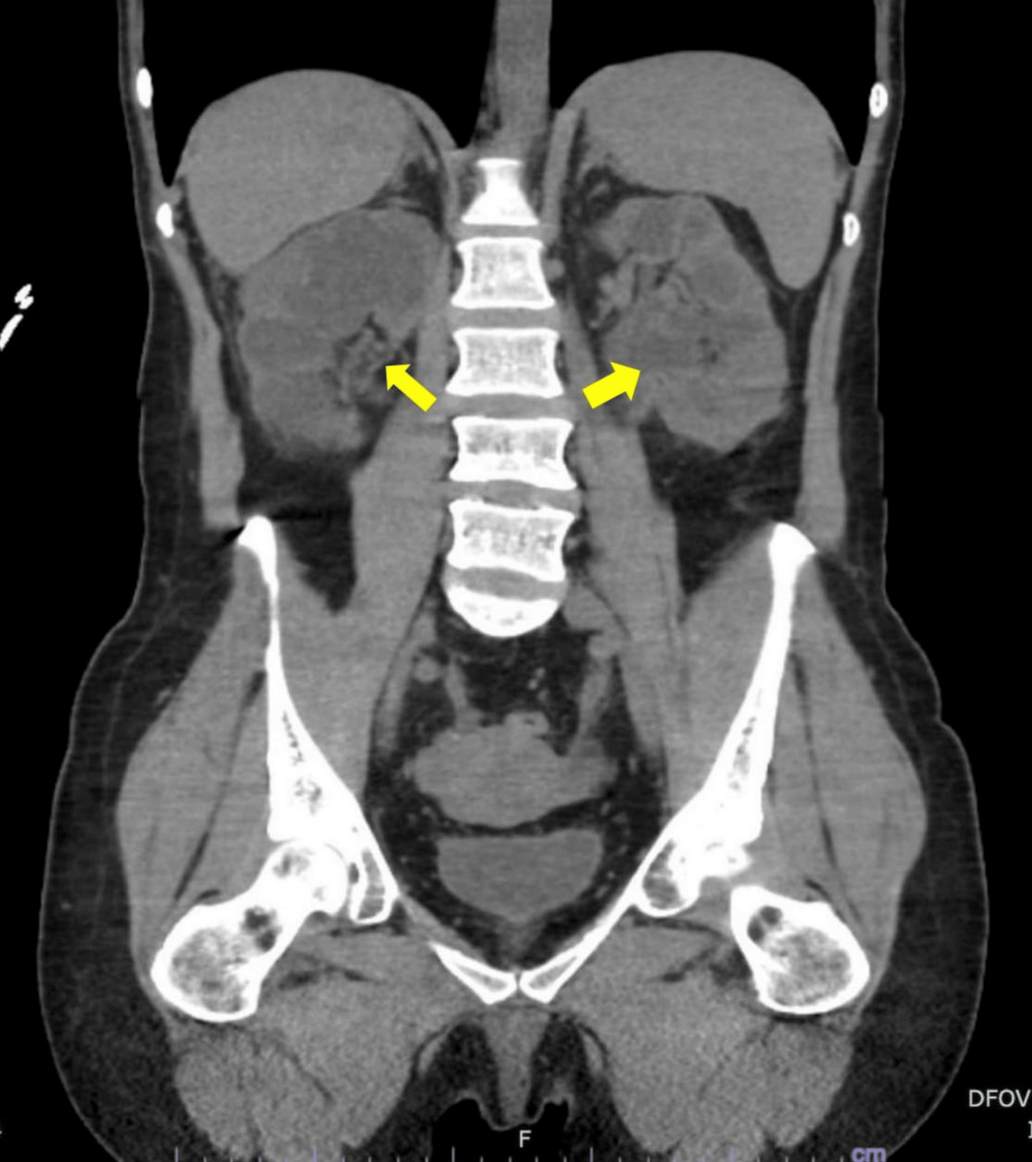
A coronal view of CT abdomen pelvis without IV contrast showing hydronephrosis before stenting.
